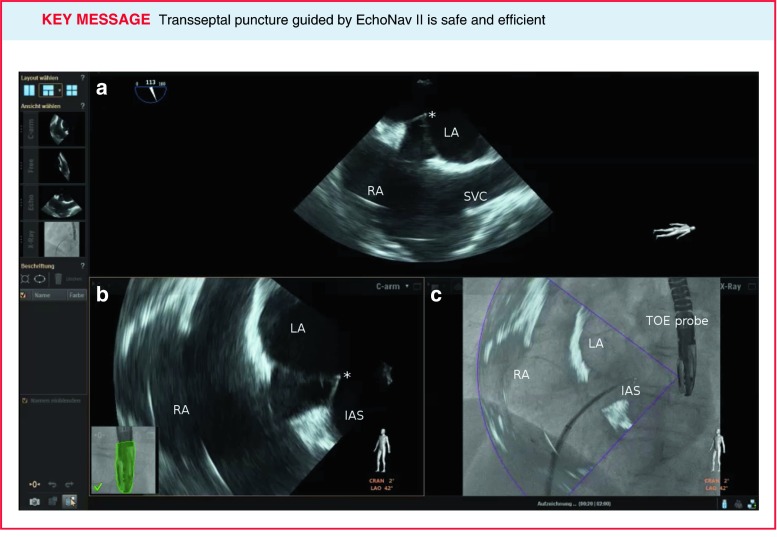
Transseptal puncture is depicted during LAA closure guided with EchoNav II. Transoesophageal echocardiogram in bicaval view shows tenting (*) of the interatrial septum (a); b reflects the calibration with the TEE probe and the 2D TEE image in the same anatomical direction as the C arm; c displays real-time fusion of echocardiographic and fluoroscopic images. *AV* aortic valve, *IAS* intra-atrial septum, *LA* left atrium, *RA* right atrium, *SVC* superior vena cava
